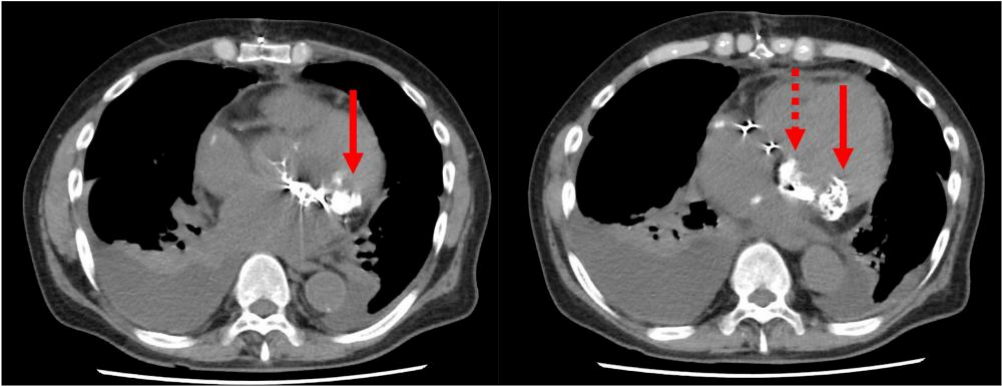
Computed tomography images after the first surgery. Left: Residual calcification (arrow) is visible outside the prosthetic valve. Right: Residual calcification is observed lateral (solid arrow) and posterior caudal (dashed arrow) to the prosthetic valve.
